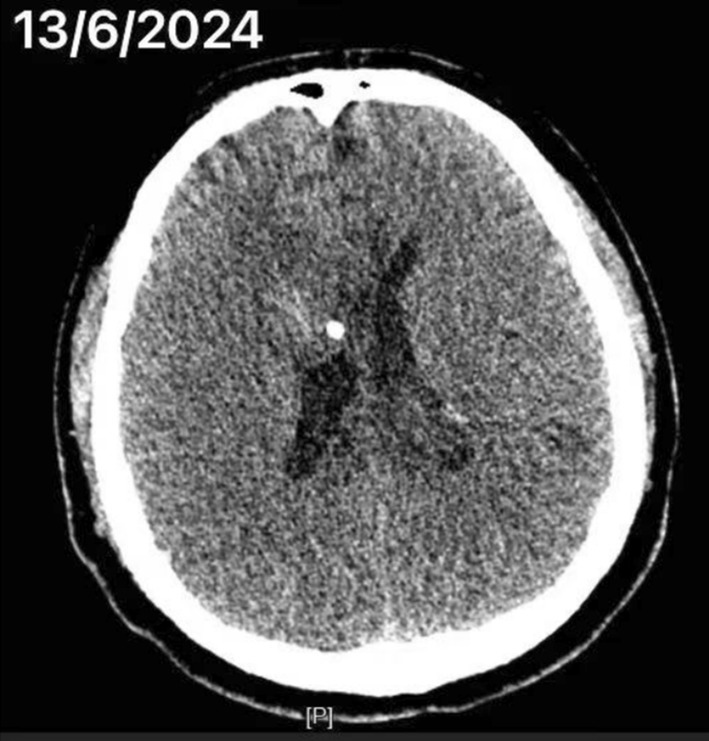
CT of the head on June 13, 2024.
